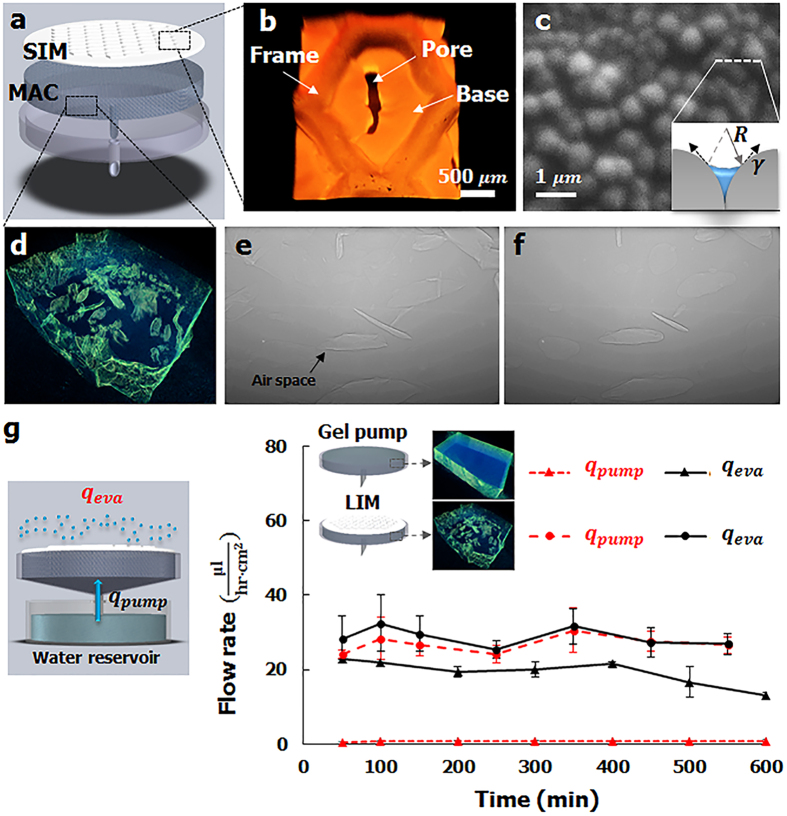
Components of the LIM and its pumping performance at room temperature. (a) The LIM is composed of two parts, namely, SIM and MAC. (b) Confocal image of the SIM, which consists of slit-shaped pores, a base part, and a thick frame. (c) A typical SEM image of the SIM surface. Rounded grooves of a hundred-nanometer scale were formed. Water molecules accumulate in the grooves and the air-water interface generates negative Laplace pressure. (d) 3D structure of the macroporous MAC visualized through an X-ray micro-imaging technique. (e) Initial state of the dehydrated MAC. (f) As water was supplied to the MAC, air spaces inside the gel were rapidly filled with water. (g) Comparison of the evaporation flow rates and pumping flow rates of the agarose gel pump and the LIM.
